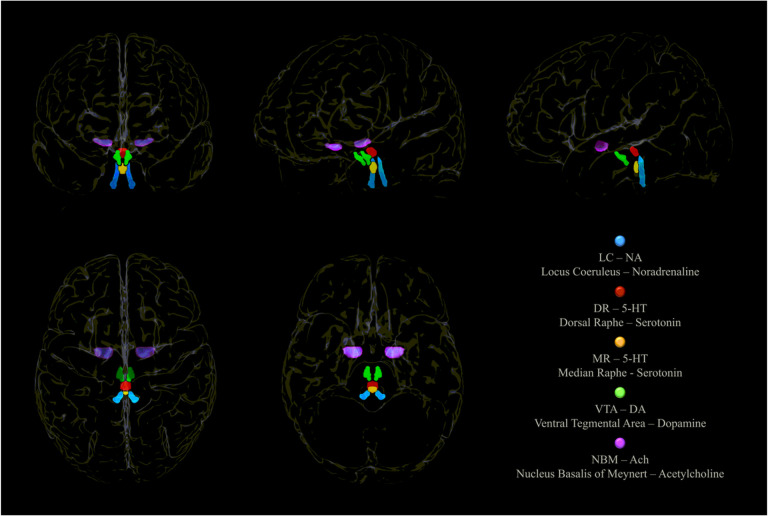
The neuromodulatory subcortical system: the main nuclei synthetizing and projecting to the cortex the main neuromodulators involved in cognition. The gure shows a 3D MRI reconstruction of the five neuromodulators’ seeds within the whole brain.
